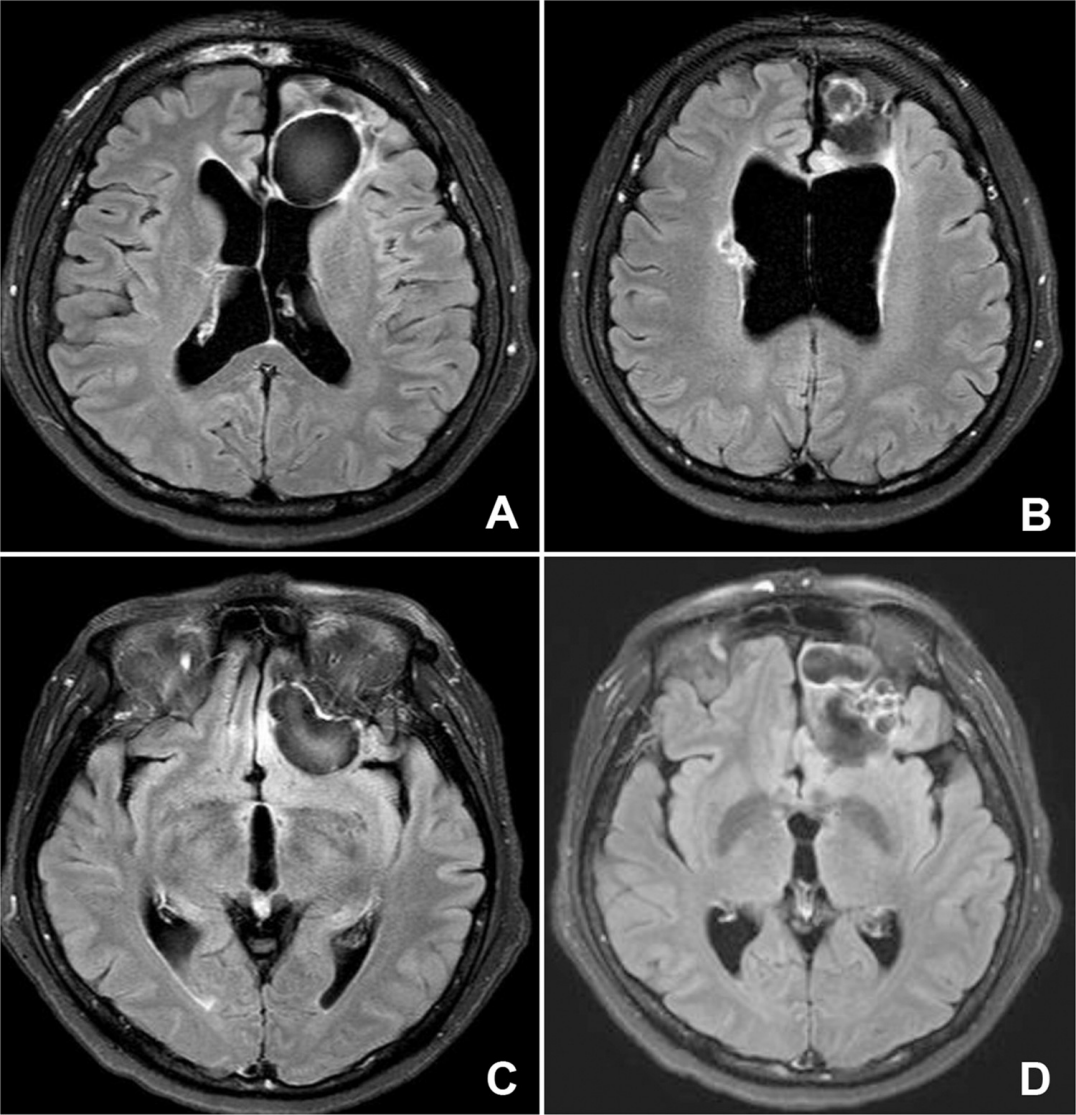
Fluid attenuated inversion recovery (FLAIR) sequence of brain magnetic resonance image of a 68-year-old female patient who suffered from dysarthria and right-sided weakness for 9 years. The laboratory results including eosinophil counts were within the normal range. The patient could not recall the consumption of freshwater crustaceans. The anti-*Paragonimus* specific IgG antibody levels against adult worm extracts were 0.31 (serum) and 0.26 (CSF), respectively. (A and B) FLAIR sequence shows cerebromalacia and ventriculomegaly with a round calcified mass on the left frontal lobe. (C and D) Several well-defined calcified masses in the left inferior frontal lobe are seen.
